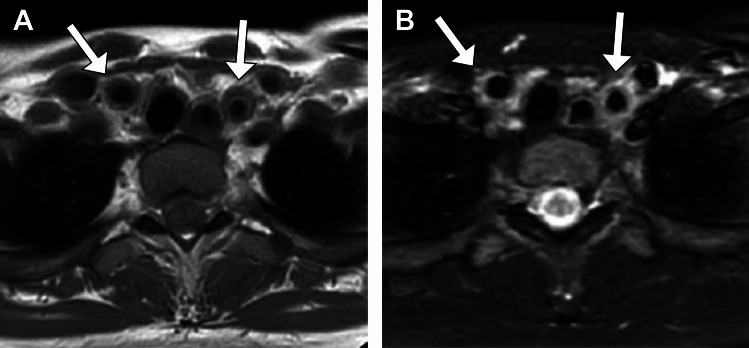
MRI in an 18-year-old woman with Takayasu arteritis. (A) T1WI and (B) STIR show wall thickening of the left and right common carotid arteries with increased signal of the wall and surroundings on STIR (B) (arrows)
